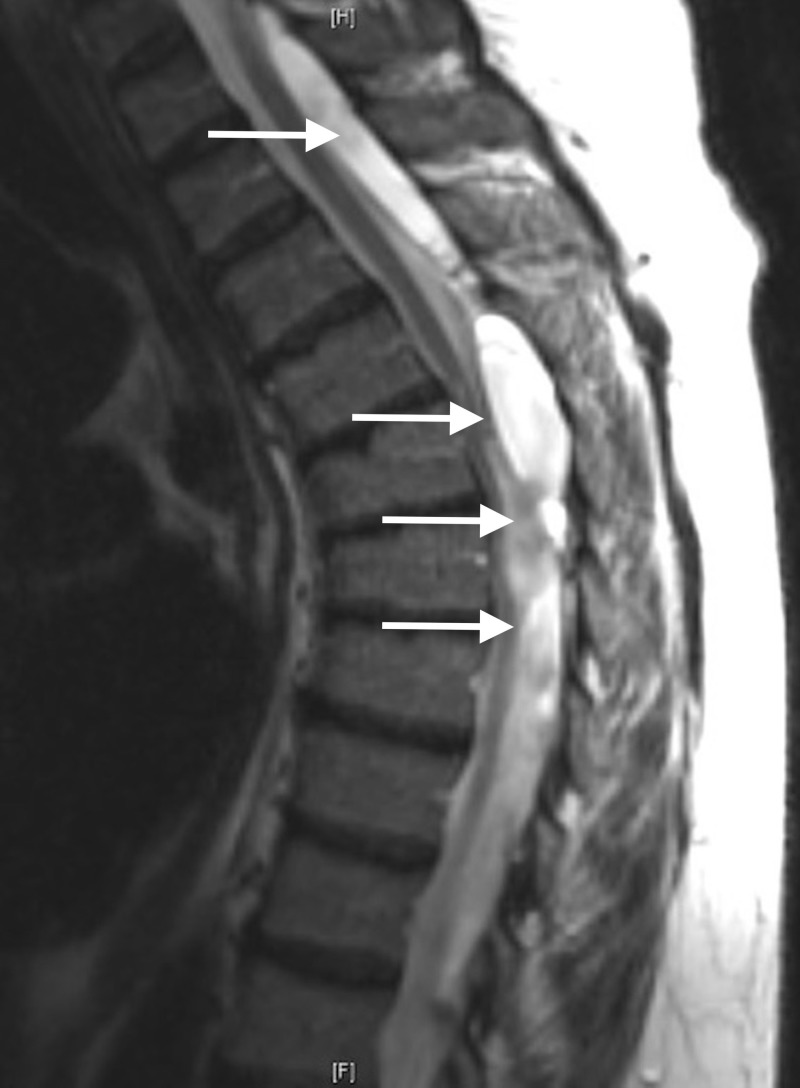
Sagittal T2 TSE MRI of the Thoracic Spine TSE: turbo spin echo; MRI: magnetic resonance imaging
